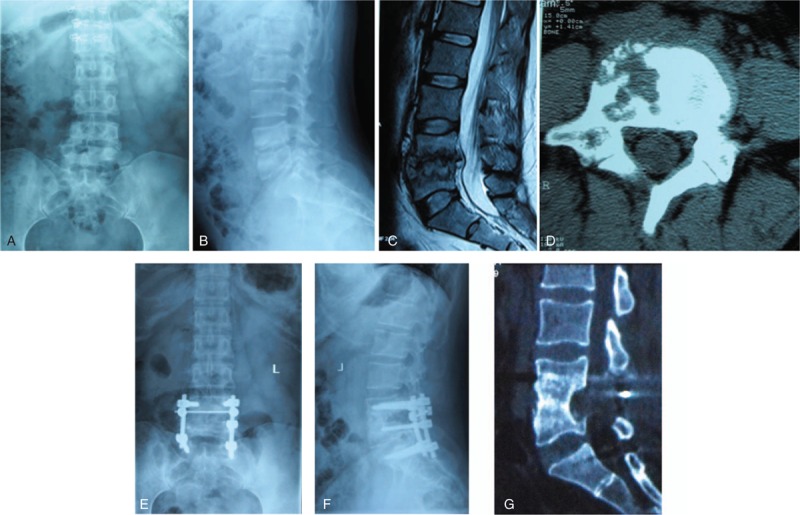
A 32-year-old female patient was diagnosed as spinal tuberculosis at the segments of L4 to L5 with paravertebral abscess. (A and B) Preoperative X-ray films of the lumbar spine. (C) Preoperative computed tomography (CT) shows vertebral destruction. (D) Preoperative magnetic resonance imaging sagittal plane shows L4 and L5 vertebral bodies bone lesion and paravertebral abscess. (E and F) The patient underwent one-stage posterior debridement with nonstructural autogenous bone grafting and instrumentation. (G) The CT scan at 12 months follow-up visit, which shows successful interbody bony fusion.
